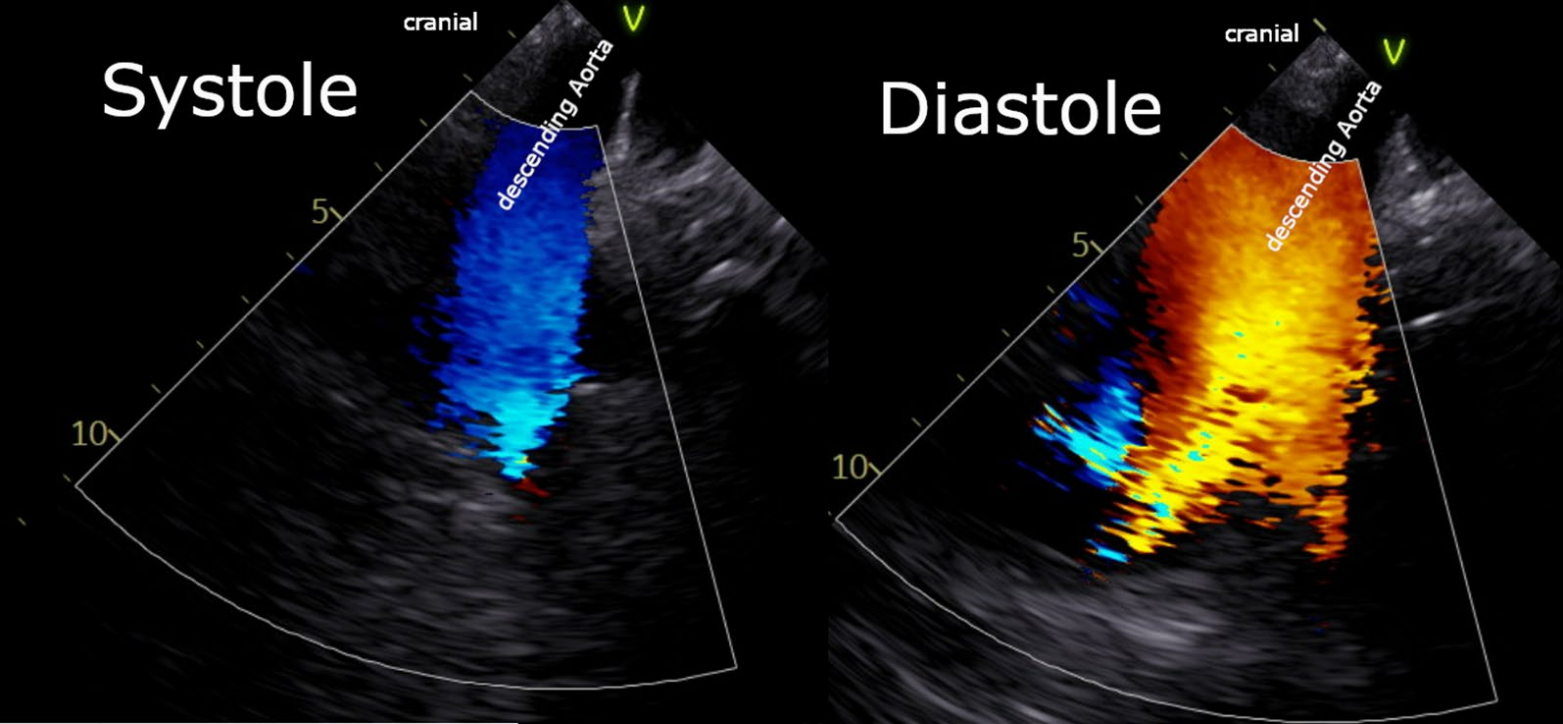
Preoperative Transesophageal Echocardiogram demonstrating significant flow reversal in the descending aorta during diastole (Right) as contrasted with the smooth systolic flow (Left)
